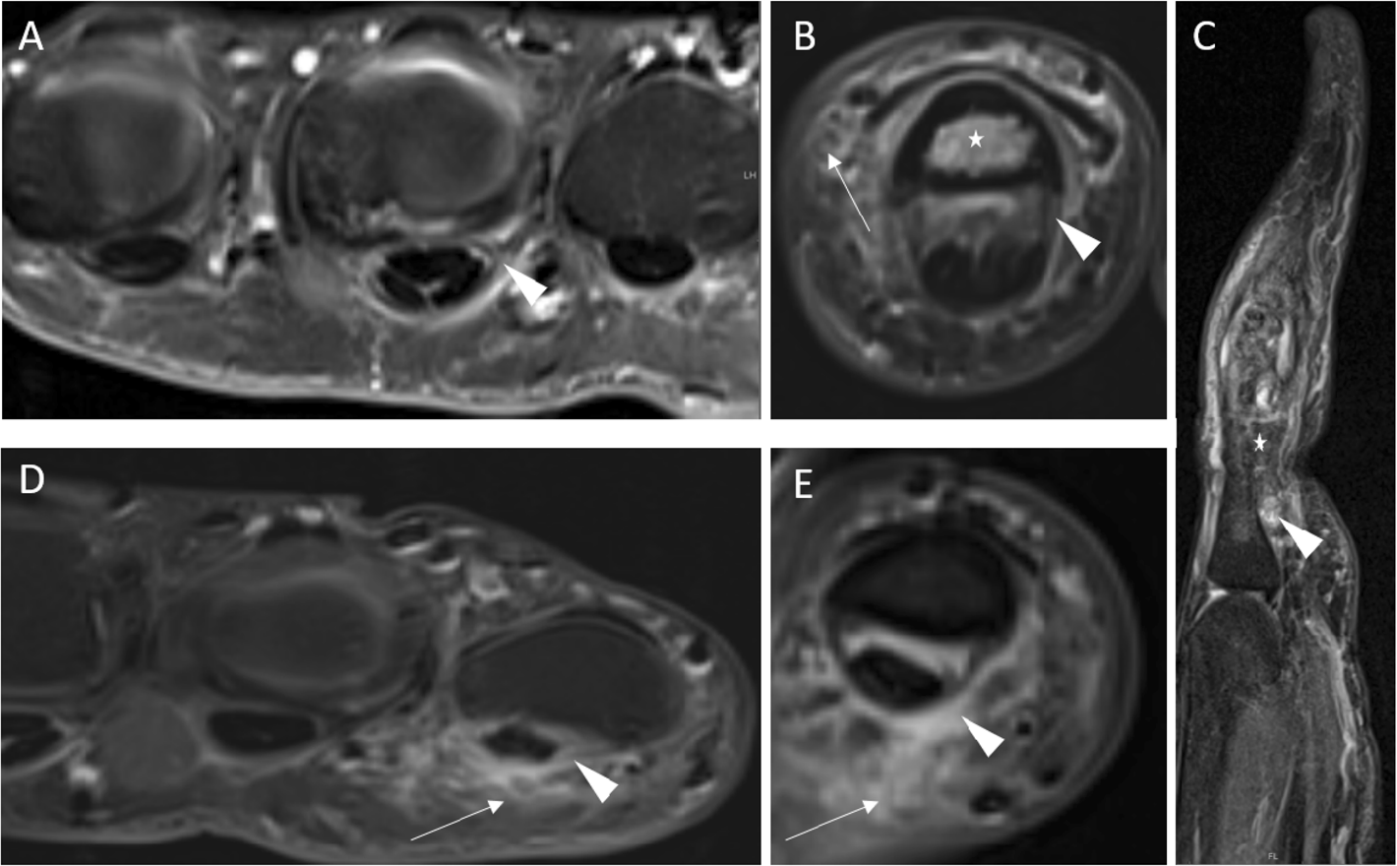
High-resolution MRI of the Hand - Figure 3
